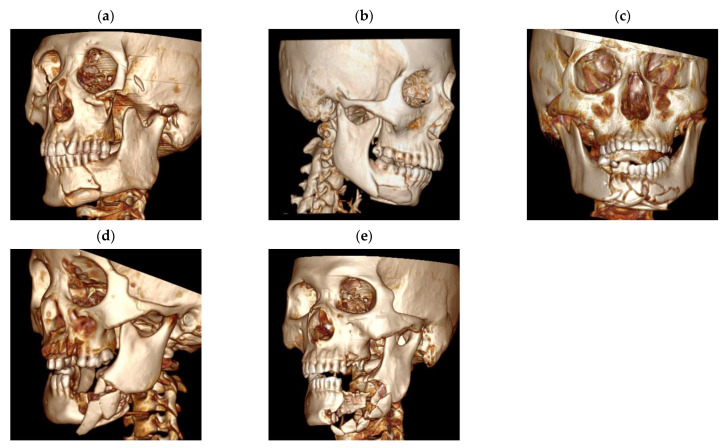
3DCT reconstruction of the OCCS classification of CMFs. (a) type I; (b) type II; (c) type III; (d) type IV; (e) type V; 3DCT: three-dimensional computed tomography; CMFs: comminuted mandibular fractures.
